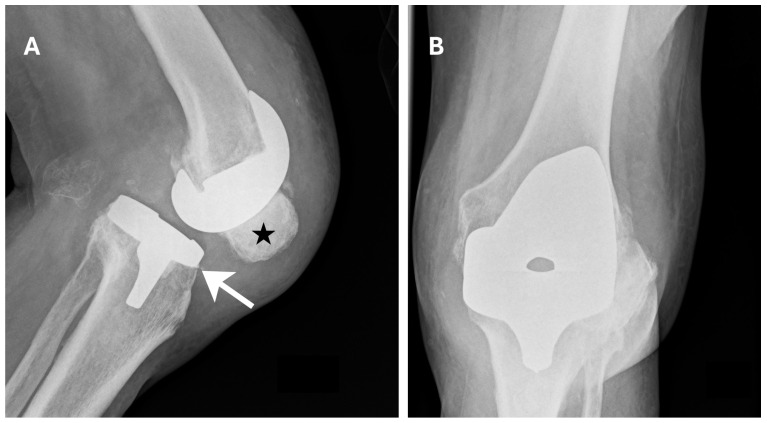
Postoperative X-ray seven months after TKA—left knee dislocation. (A) Lateral plane. (B) Anteroposterior (AP) plane. The lateral view shows joint instability in the form of posterior tibial dislocation (white arrow). Note the low position of the patella (black asterisk). The prosthetic components show no obvious signs of loosening.
